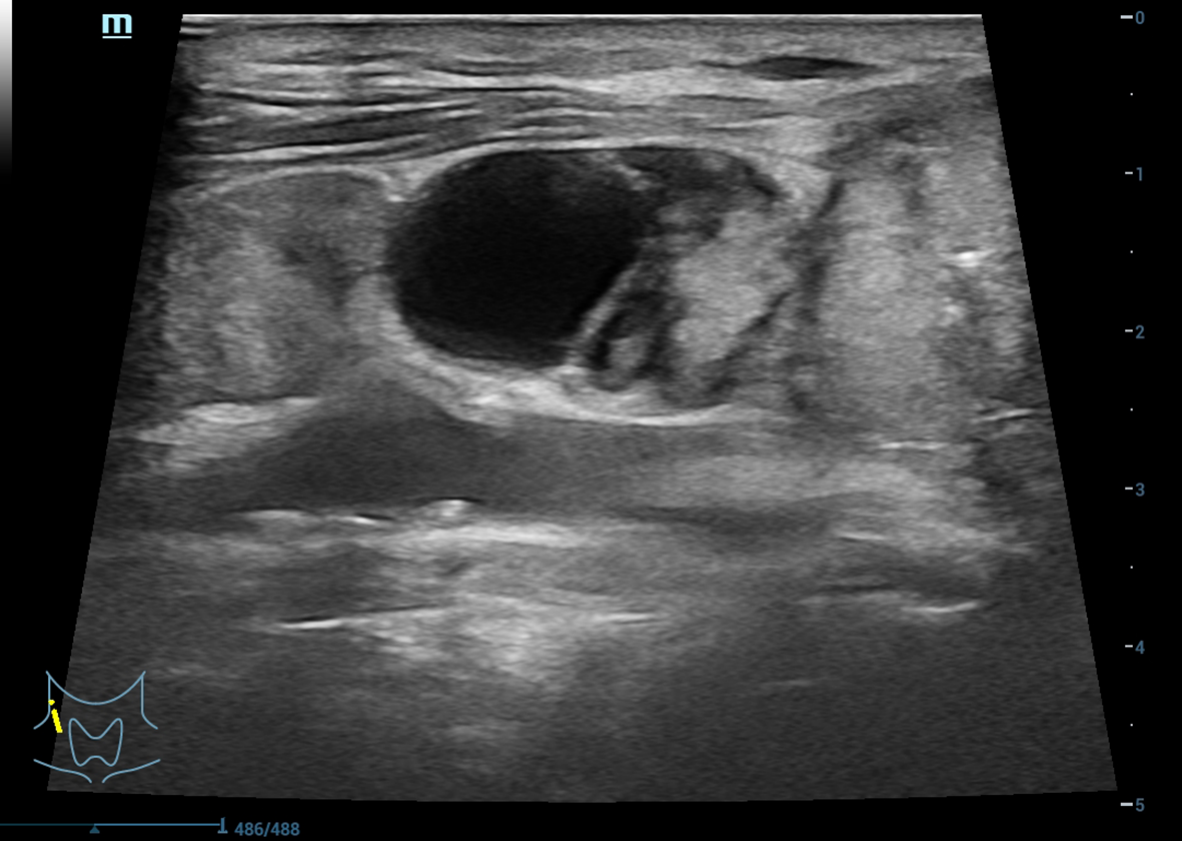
Metastatic lymph nodes accompanying internal liquefactive necrosis in right neck as shown in ultrasonography.
